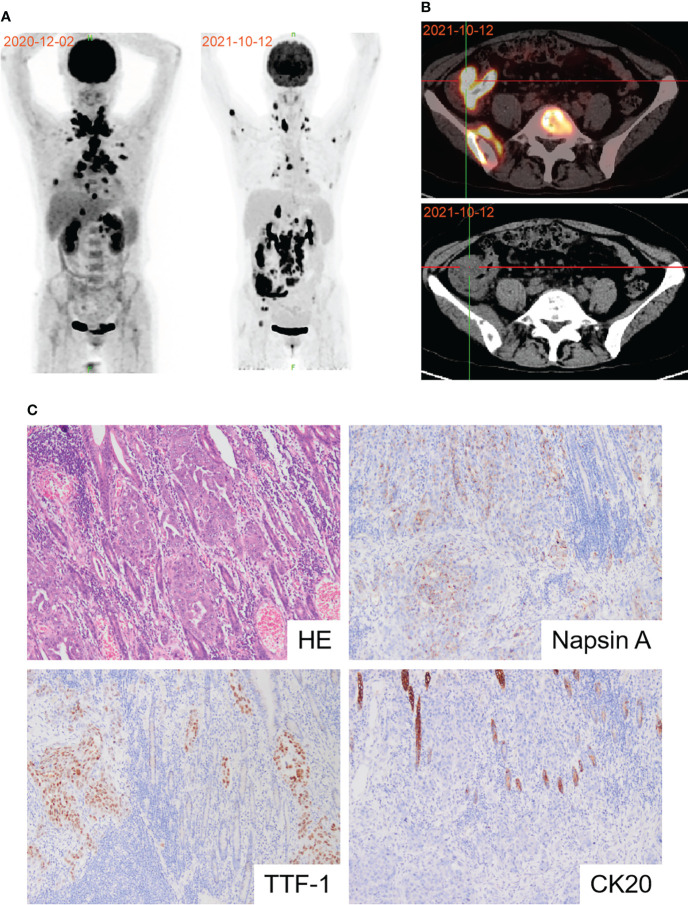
Diagnosis of colon metastasis from lung adenocarcinoma. (A) PET-CT scan showed the effects of first-line immunochemotherapy. (B) Representative PET-CT image of colon metastasis. (C) Pathological image of postoperative colon mass notarized colon metastasis of lung adenocarcinoma.
